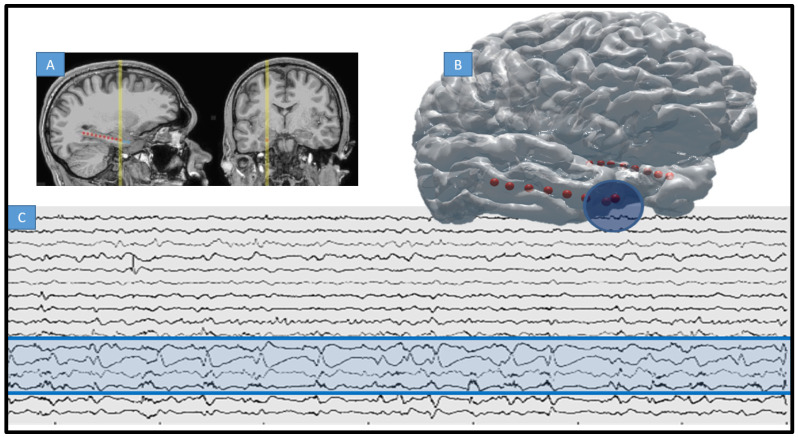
Intracranial EEG and MRI-CT co-registered electrodes. (A) T1 showing location of 4 macroelectrode contacts implanted along the hippocampus of a patient with MTS and MRE of temporal lobe (arrow points to electrode trajectory indicating occipital approach). (B) 3D rendering of typical electrode placement within same patient, red indicating macro contact of depth and blue the particular SOZ for this patient (first four electrodes of right depth, or anterior temporal pole). SOZ = seizure onset zone. (C) Raw intracranial EEG trace for same patient are recorded on 8 depth electrodes as in (A) (SOZ marked in blue circle and blue shading on raw EEG).
